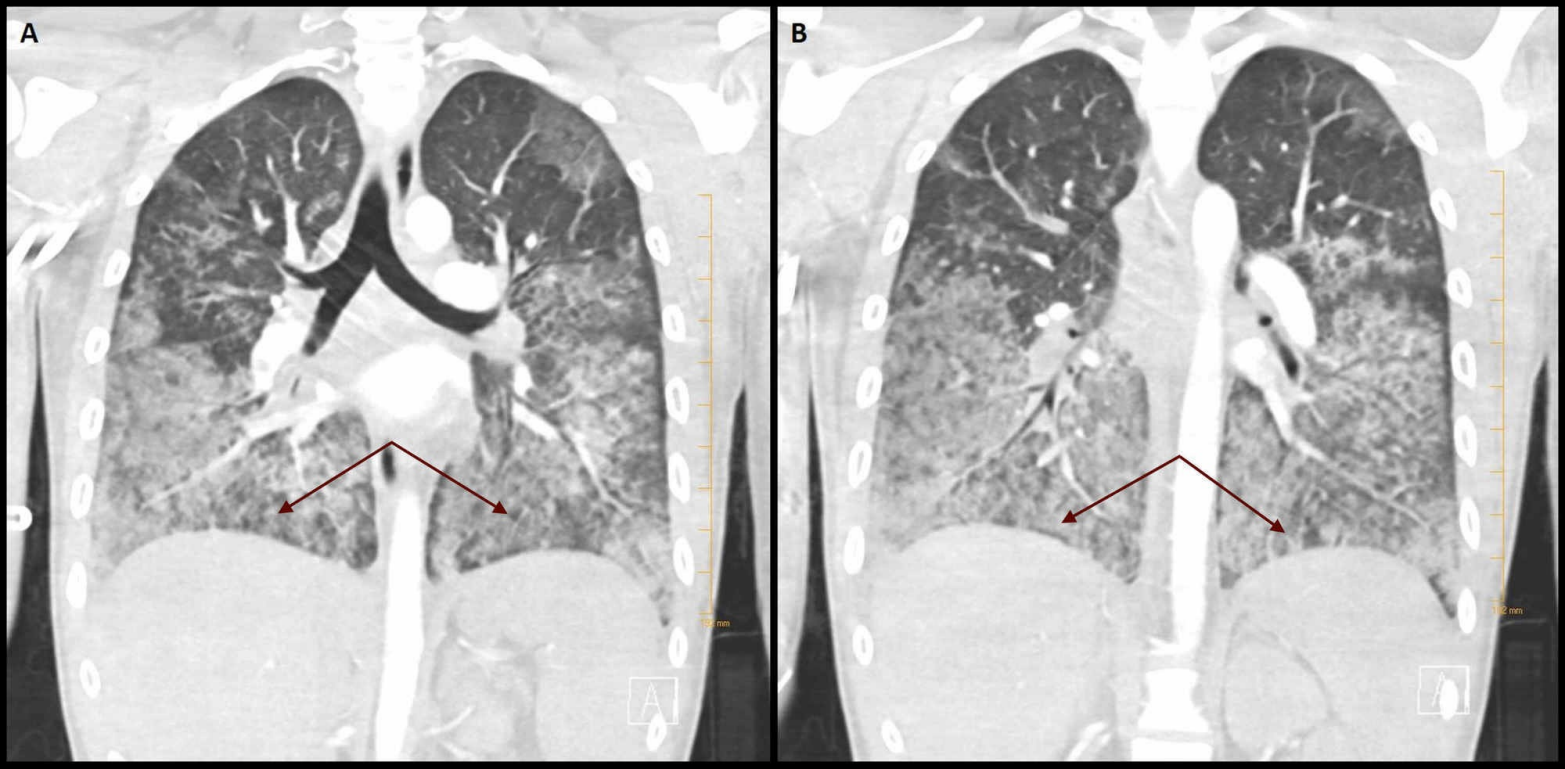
Computed tomography of the chest. (A, B) Coronal section of computed tomography of the chest (arrows) demonstrating bilateral lower lobe predominant ground-glass opacities.
